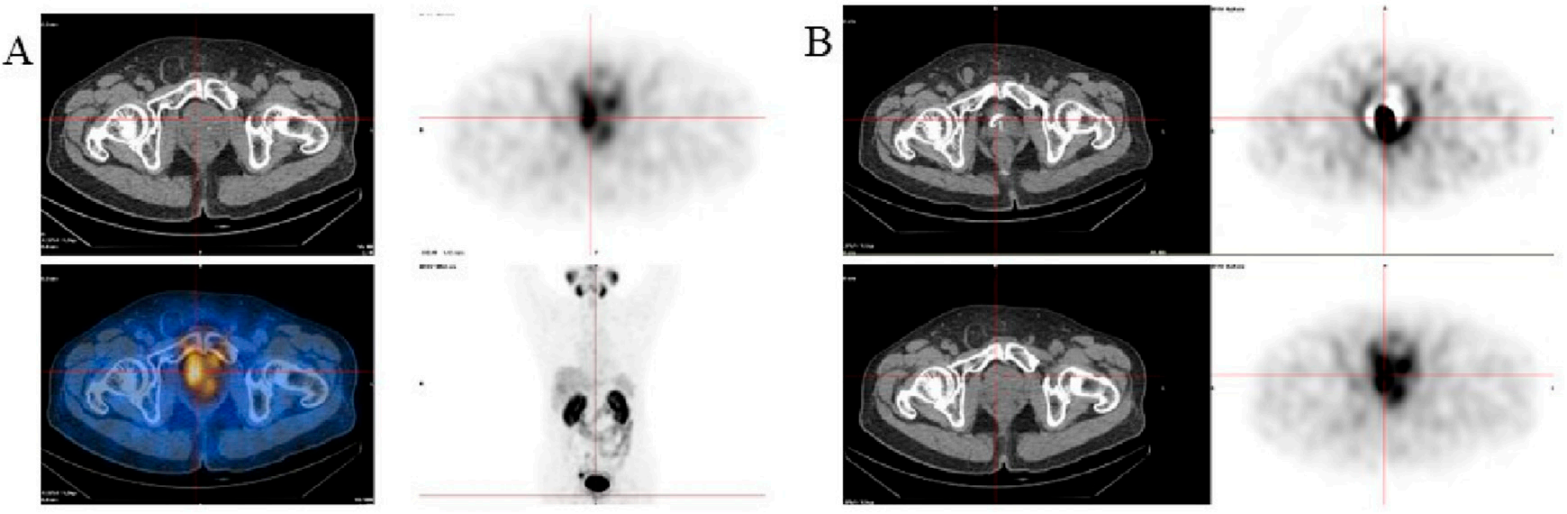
Representative PSMA images of a patient receiving darolutamide-based triplet therapy followed by high-dose intensity-modulated radiotherapy (IMRT). (A) PSMA IMAGING after completion of triplet therapy showing residual focal uptake within the prostate, indicating partial metabolic response after systemic treatment. (B) Follow-up PSMA IMAGING 3 months after high-dose IMRT demonstrating a marked reduction in SUVmax and metabolic activity of the primary lesion, consistent with continued PSA decline and durable local control.
